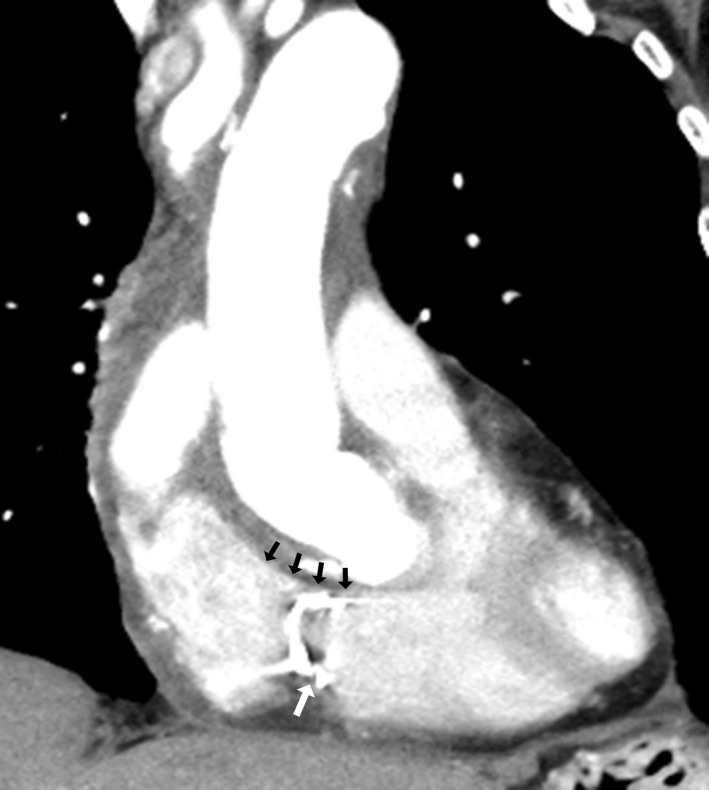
Postoperative contrast‐enhanced chest computed tomography. The right heart returns to a normal contour (black arrows), and the tricuspid annulus is repaired with a prosthetic annulus (white arrow).
